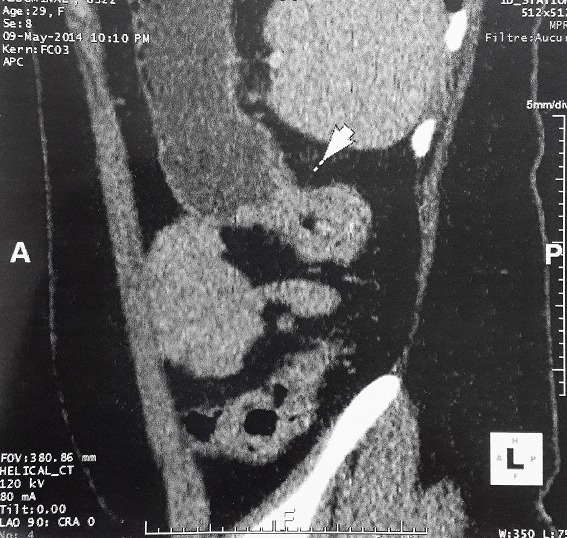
Abdominal CT scan showing irregular parietal thickening of the descending colon with important dilation of the colon upstream
